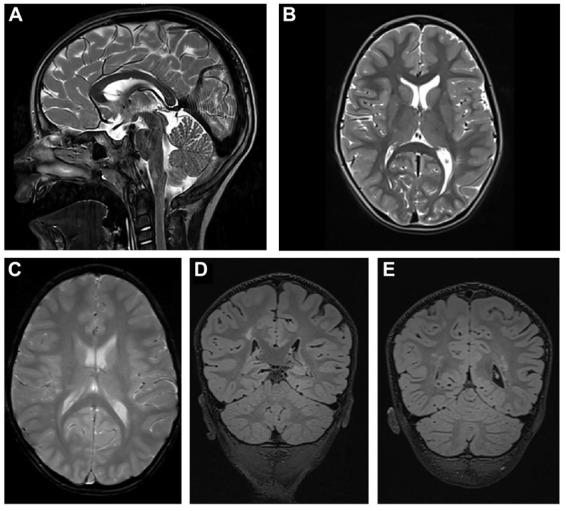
Cerebral MRI. (A) T2-weighted sagittal TSE image of the patient shows a slightly thinned but fully formed corpus callosum and normal cerebellar structures. (B) T2-weighted axial TSE image of the patient reveals normal gyral pattern of the cerebral cortex and normal volume of white matter but delayed myelination of the internal capsules. (C) T2-weighted axial GRE image (T2^∗^) shows diffuse white matter abnormalities in the periventricular and subcortical. (D,E) T2-weighted coronal 3D-FLAIR images demonstrate focal as well as diffuse areas of hyperintensity in periventricular white matter.
